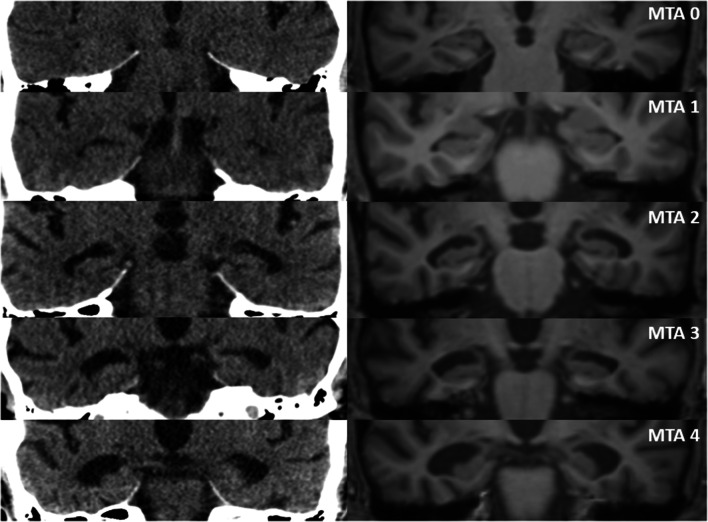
Image examples from MTA grades according to Scheltens’ ordinal 5-point MTA scale (CT to the left). Each side was rated separately, and the overall highest score was given. MTA 0 = no atrophy, MTA 1 = mild widening of choroid fissure, MTA 2 = moderate widening of choroid fissure *and* mild widening of temporal horns *and* mild decrease in hippocampal height, MTA 3 = severe widening of choroid fissure *and* moderate widening of temporal horns *and* moderate decrease in hippocampal height, and MTA 4 = severe widening of choroid fissure *and* severe widening of temporal horns *and* severe decrease in hippocampal height. Abbreviations: MTA, medial temporal lobe atrophy
